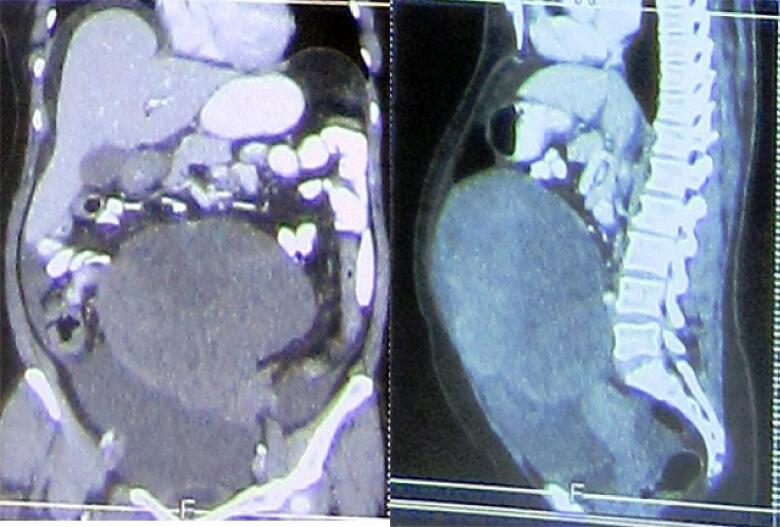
Abdomino-pelvic CT scan, longitudinal and axial view.
